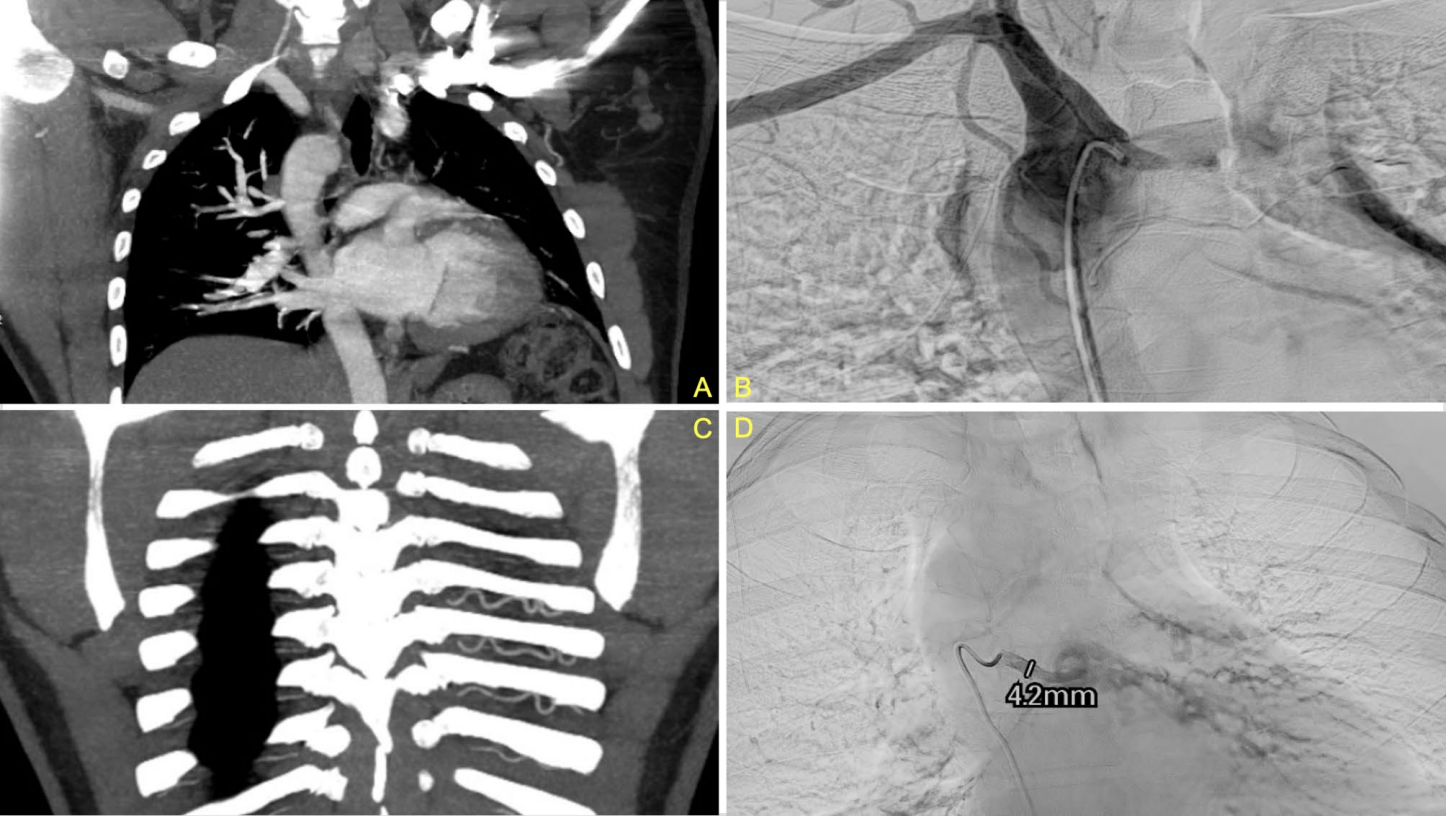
Computed tomography of right-sided aortic arch (2a). The patient demonstrated a variant arch vessel branching pattern with a left brachiocephalic artery as the first branch (not pictured), a right common carotid artery as the second branch, and a right subclavian artery as the third branch (2b) as well as hypertrophy of the intercostal and bronchial arteries (2c and 2d)
